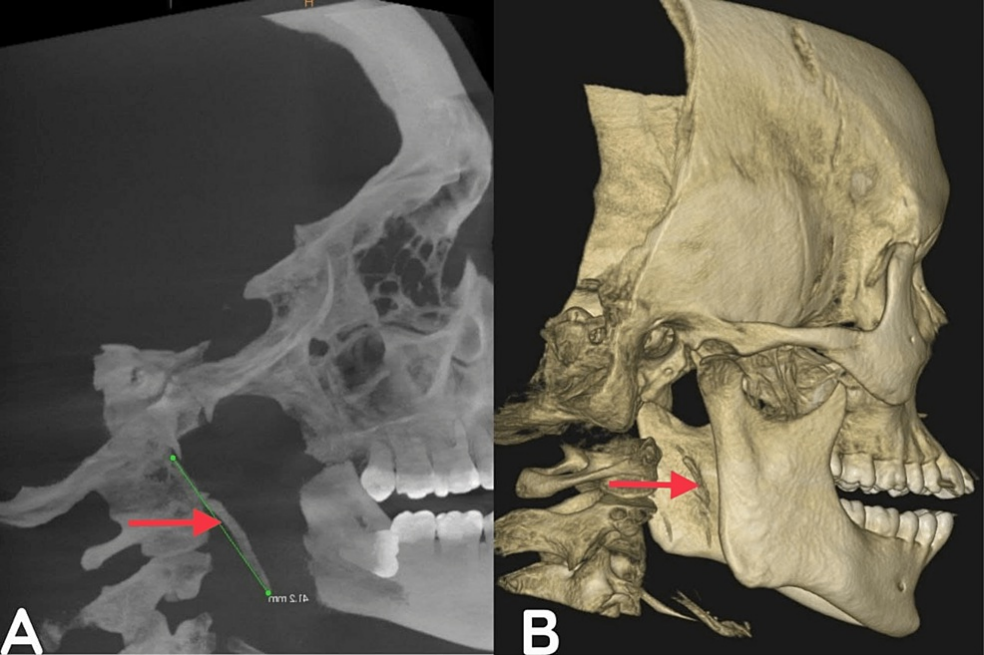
Pre-operative CBCT The arrow mark shows the elongation of the styloid process in the transverse view (A) and 3-dimensional view of CBCT (B)
